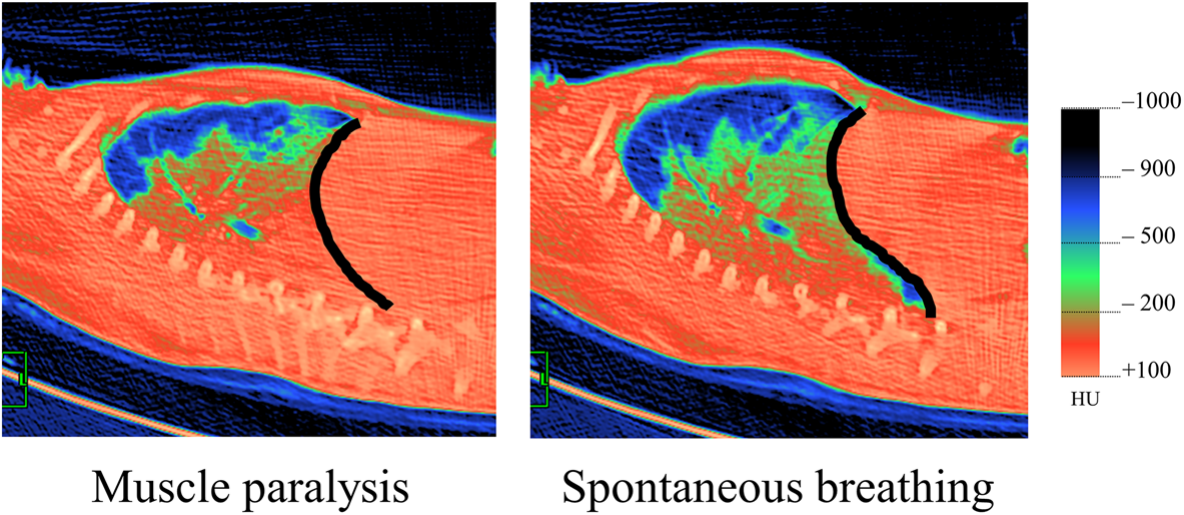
CT images in experimental lung injury—muscle paralysis vs. spontaneous breathing. Both dynamic CT images of the same anatomical, sagittal level at end-expiration are shown to compare the end-expiratory lung volume and the shape of the diaphragm. CT images are colored according to their Hounsfield units densities. The black lines indicate the diaphragm at end-expiration. These dynamic CT images were continuously taken after injection of neuromuscular blocking agent, without any change in ventilatory settings. Spontaneous breathing effort restored the end-expiratory lung volume due to diaphragmatic muscle tone. Once diaphragm was paralyzed, diaphragm shifted to cranial direction, resulting in large collapse in dorsal lung regions. Note that this happened because inadequate (low) PEEP was applied.
